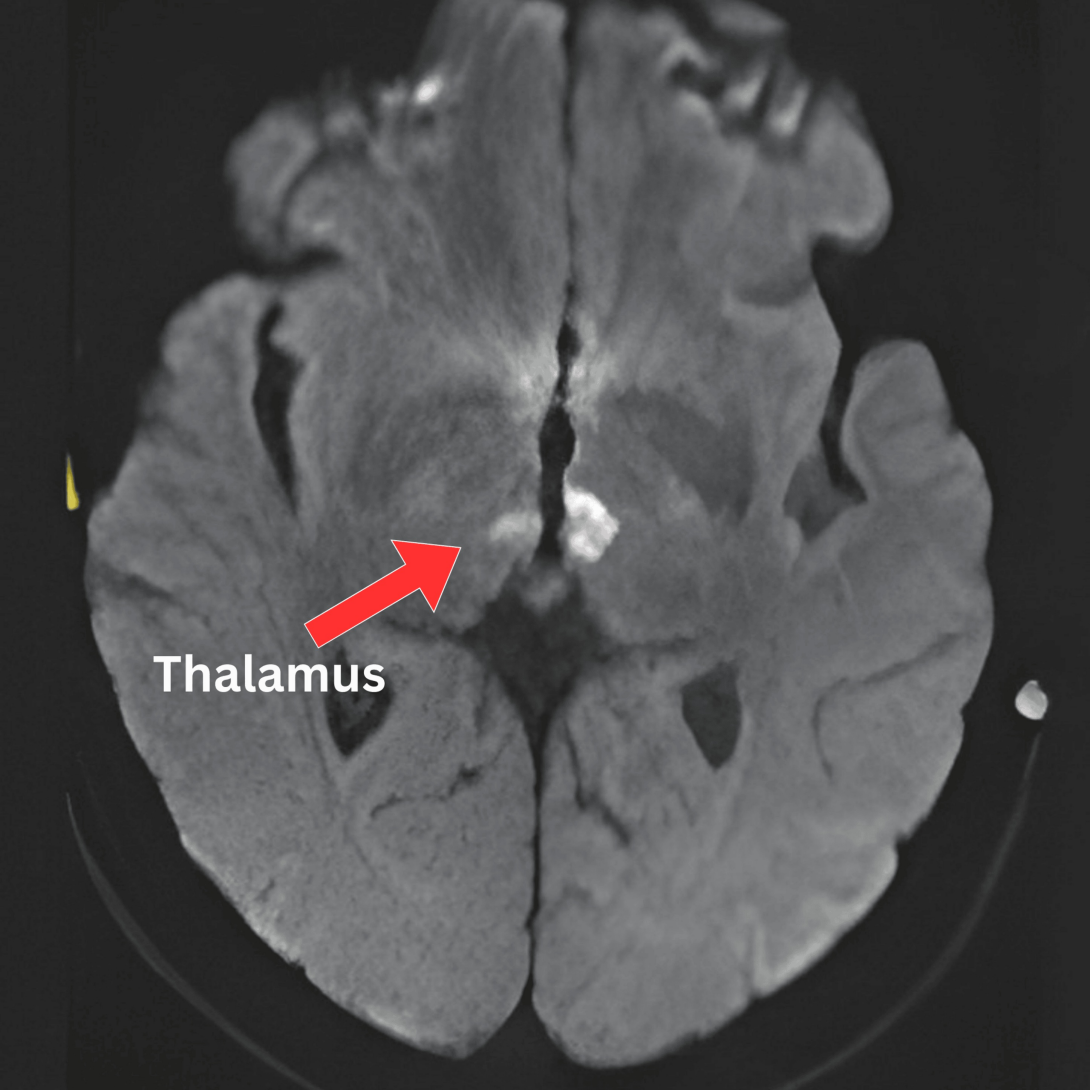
Non-contrast MRI brain DWI sequence showing corresponding diffusion restriction within bilateral thalami, consistent with cytotoxic oedema. DWI: diffusion-weighted imaging; MRI: magnetic resonance imaging.
